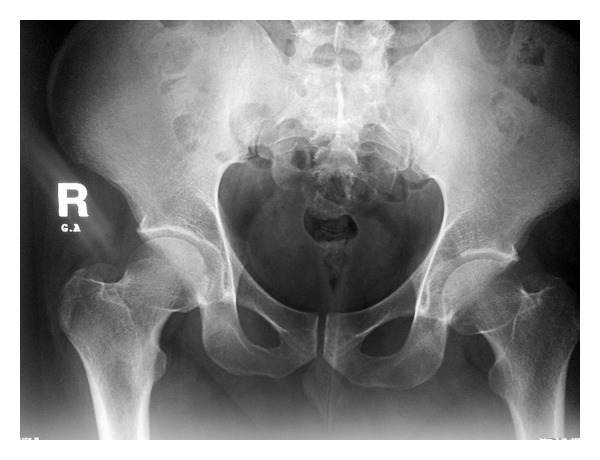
Ap Pelvis X-ray showing a lucency in the left acetabular dome.
